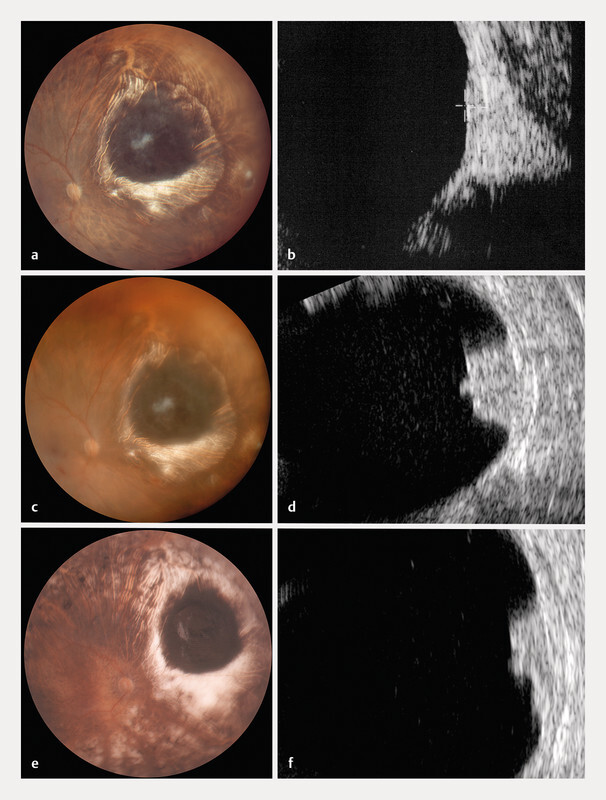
Follow-up color fundus pictures and B-scan ultrasonography.
